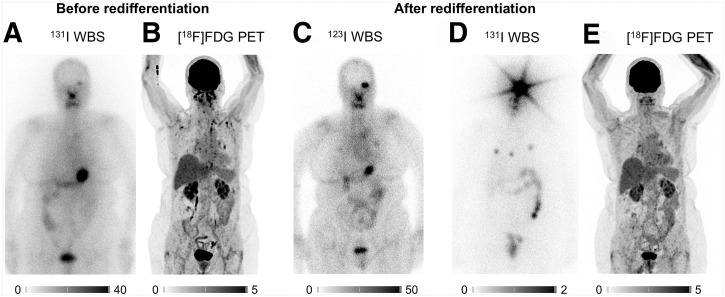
Comparison of baseline ^131^I-scintigraphy, [^18^F]FDG PET (A and B), and postinterventional ^123^I scan (C) after successful redifferentiation in patient 7. Iodine uptake increased, especially in left orbital metastasis and in left and 2 right pulmonary metastases, which led to RAIT. Depiction of whole-body scintigraphy (WBS) scan after high-dose RAIT with significant iodine uptake in newly iodine-avid lesions (D) and of [^18^F]FDG PET scan 4 mo after RAIT (E) with assessment of partial response. Intensity scales are SUV for PET and % for scintigraphic images.
